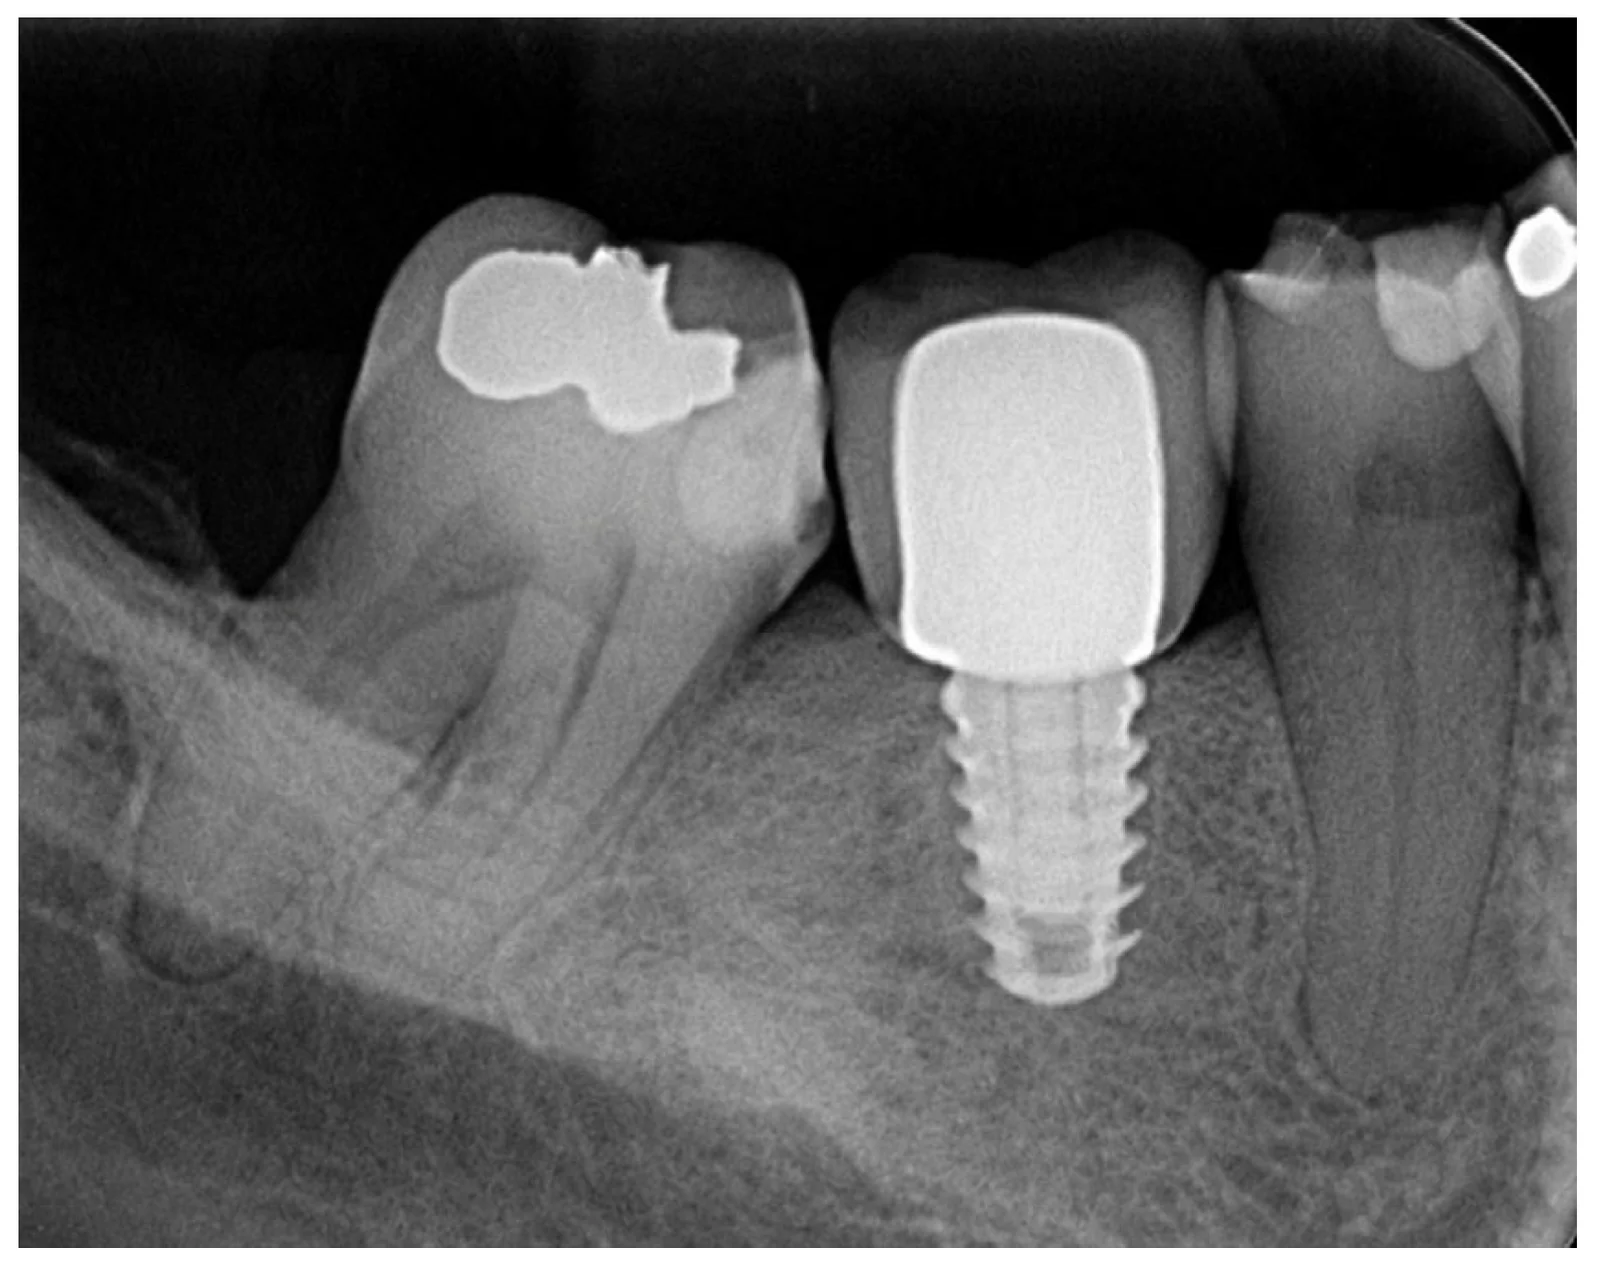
Periapical radiograph at 4-year follow-up.
